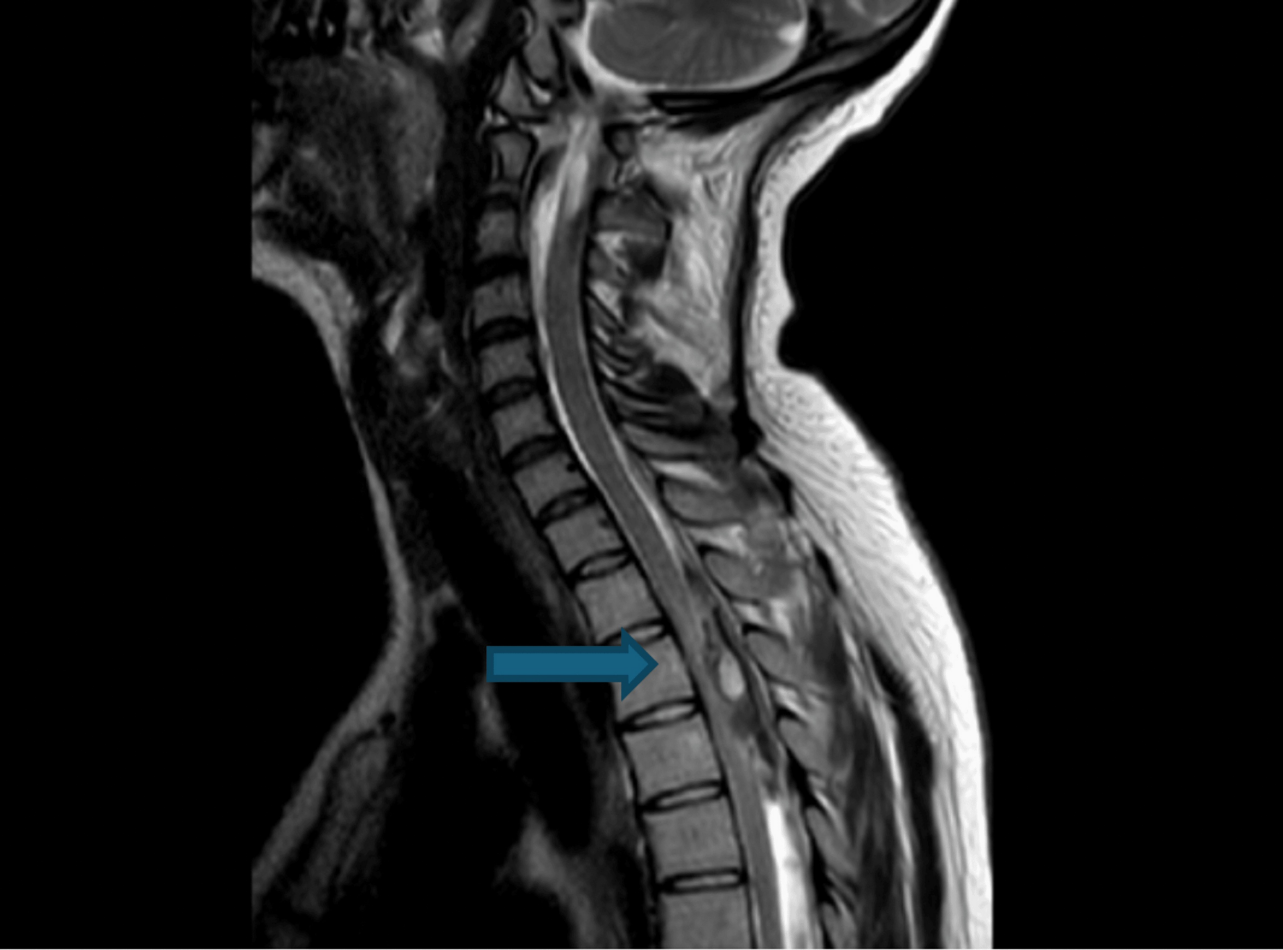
Sagittal T2-weighted MRI showing intrathecal haematoma most prominent at T3-T4. The blue arrow indicates the location of the intrathecal spinal haematoma on sagittal MRI.
